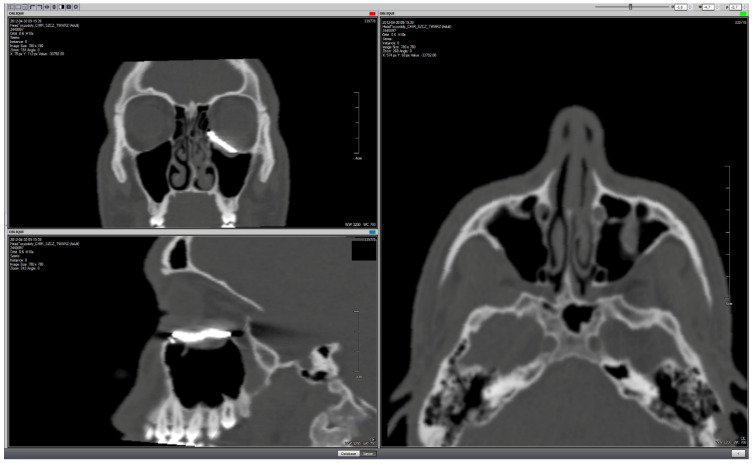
Postoperational computed tomography. Patient-specific implant reconstruction of the left orbital floor. Note: the artifacts around the implant (the real implant thickness varied from 0.46 to 0.71 mm, with a pseudo-thickness up to approx. 4 mm) caused by hardening of the X-ray beam due to the high molecular weight of the material.
